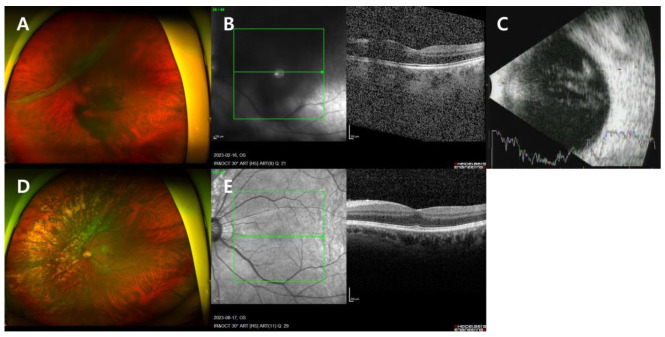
Case 1 patient’s left eye images. (A–C) On the day of presentation. (A) Color fundus photography (FP) showed rhegmatogenous retinal detachment (RRD) with giant retinal tear (GRT) at a 9–12 o’clock direction. (B) Optical coherence tomography (OCT) showed vitreous hemorrhage. (C) B-scan ultrasound (B-scan) showed vitreous hemorrhage and retinal detachment. (D,E) Six months after surgery. (D) FP showed a flat, well-attached retina. (E) OCT showed a flat, well-attached retina.
